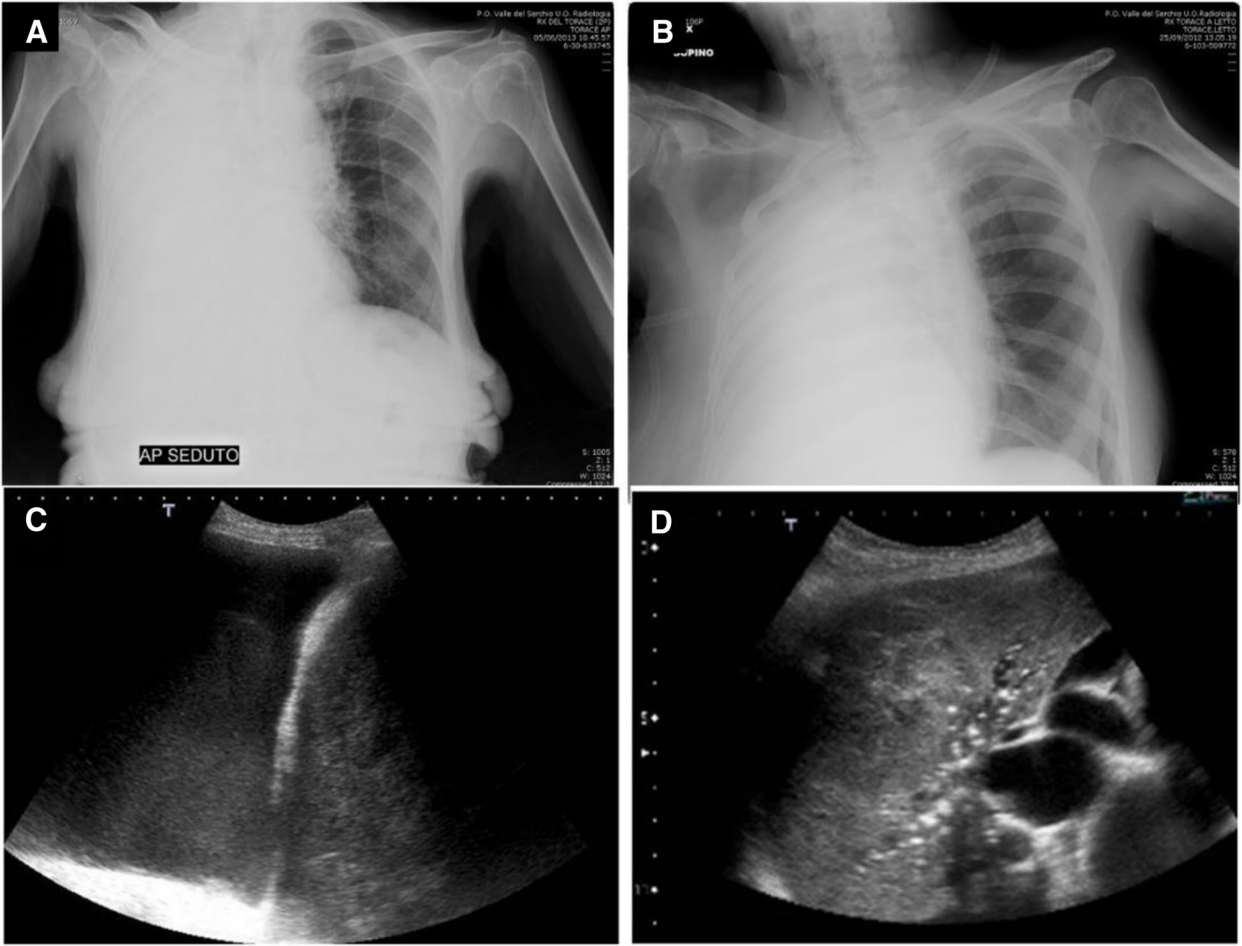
a and b: Chest x rays showing two patients with opaque right hemithorax. c and d: ultrasound scans of the right hemithorax in the same patients. The diagnosis is immediate (hyper-codified abduction). a. massive pleural effusion. b: right lung atelectasis. The right main bronchus is closed by a large hilar mass
